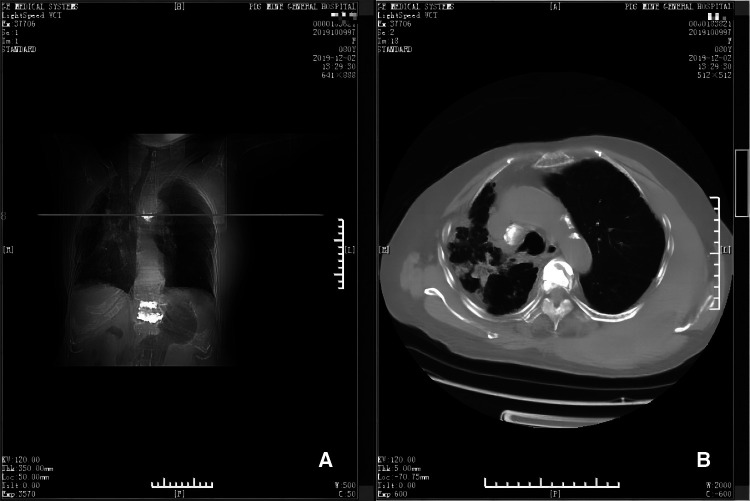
Emergency CT scans (panels A and B). Panel A depicts the position (horizontal line) of the CT scan shown in B, at the level of the 5th thoracic vertebra. Panel B shows that there is a large hematoma near the 5th thoracic vertebra and adjacent ribs.
